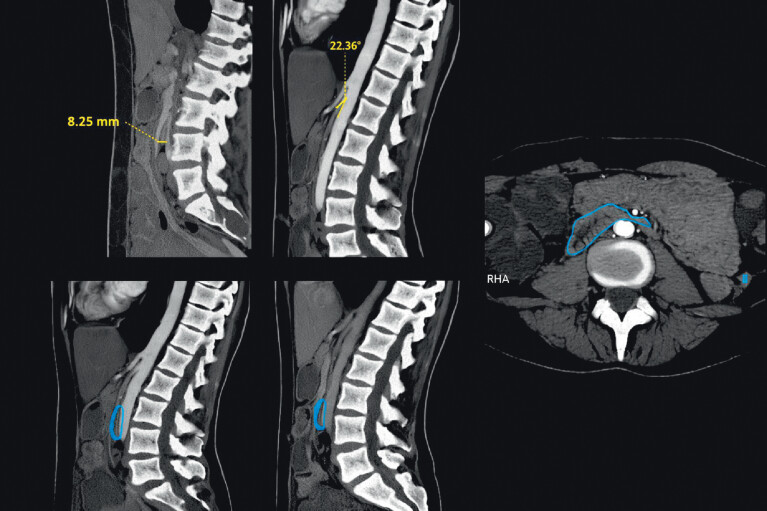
CT enterography demonstrating extrinsic compression of the third portion of the duodenum (D3) by the superior mesenteric artery, resulting in luminal narrowing and proximal stasis.
